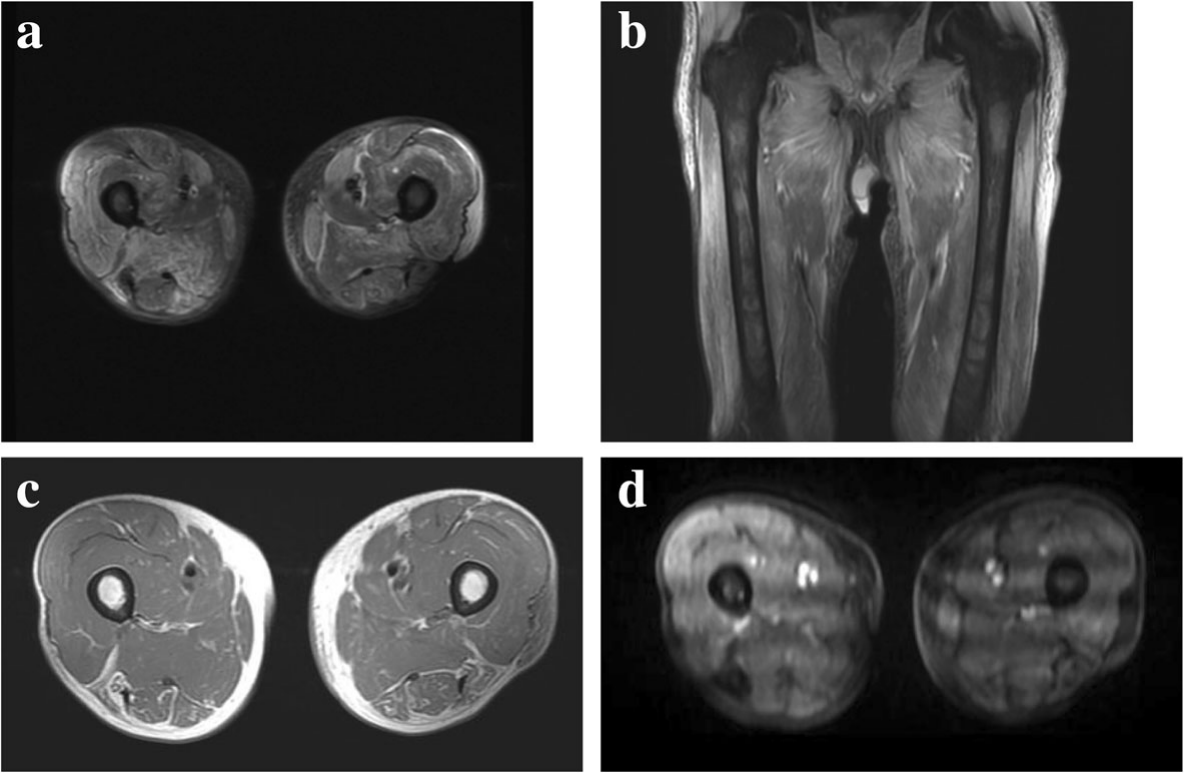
Myositis detected by magnetic resonance imaging. a, b Magnetic resonance imaging of the bilateral femoral shows a heterogeneous high signal intensity in the bilateral femoris muscles in short-T1 inversion recovery images. c T1 image before enhancement. d A heterogeneous contrast enhancement in the bilateral femoris muscles in gadolinium-enhanced T1 images
